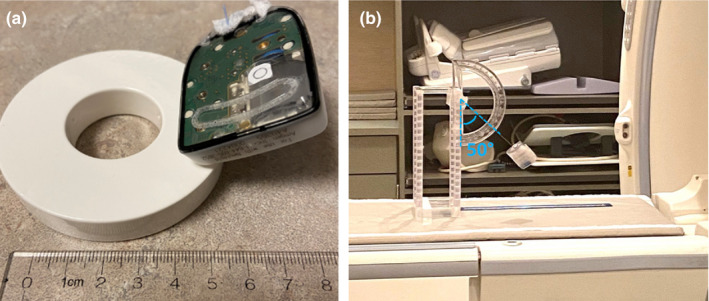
(a) Photograph of the portion of the device that showed the strongest pull force toward the hand‐held magnet. (b) The magnetically induced displacement test in Zone 4 of a 1.5T GE MRI scanner showed a deflection angle of 50 degrees.
